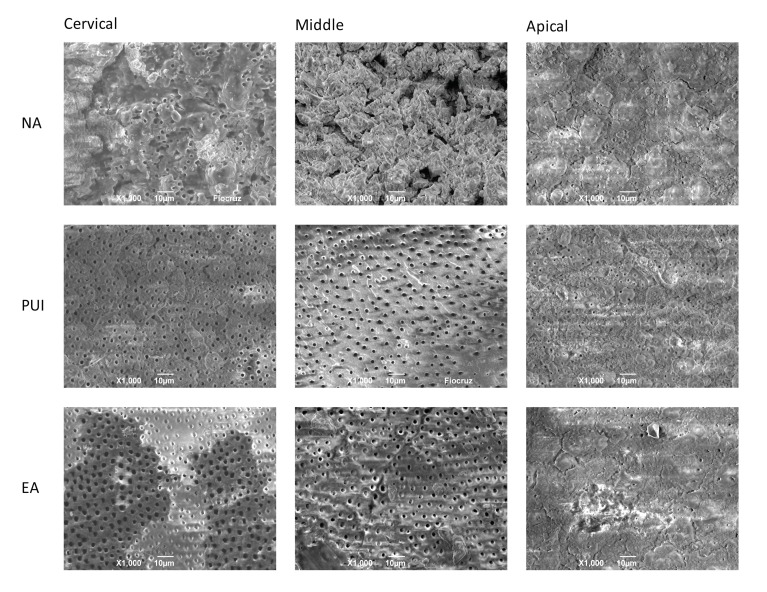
Representative SEM images of smear layer removal from different root thirds, after applying the irrigation techniques being tested. NA: Non-activated; PUI: Passive ultrasonic irrigation; EA: EndoActivator system.
